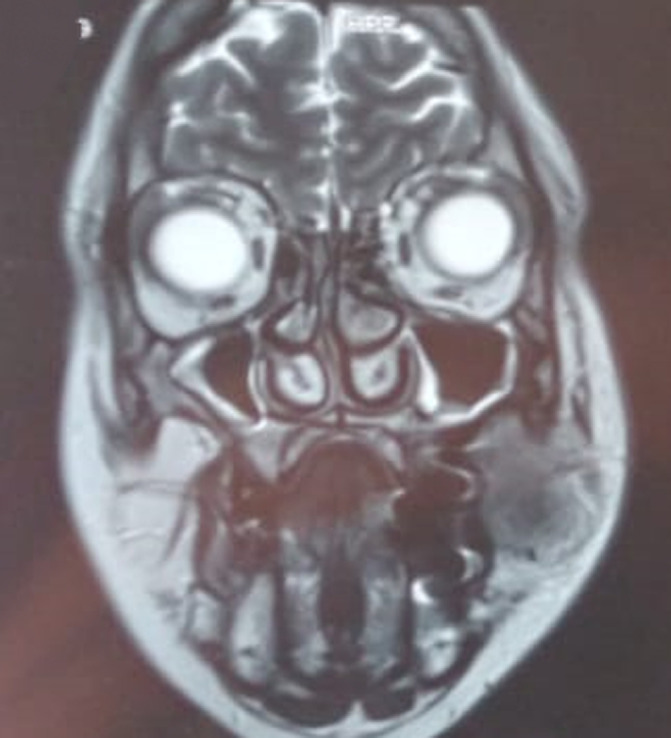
magnetic resonance imaging demonstrating osteolysis and masseter muscle infiltration in Rosai-Dorfman case
